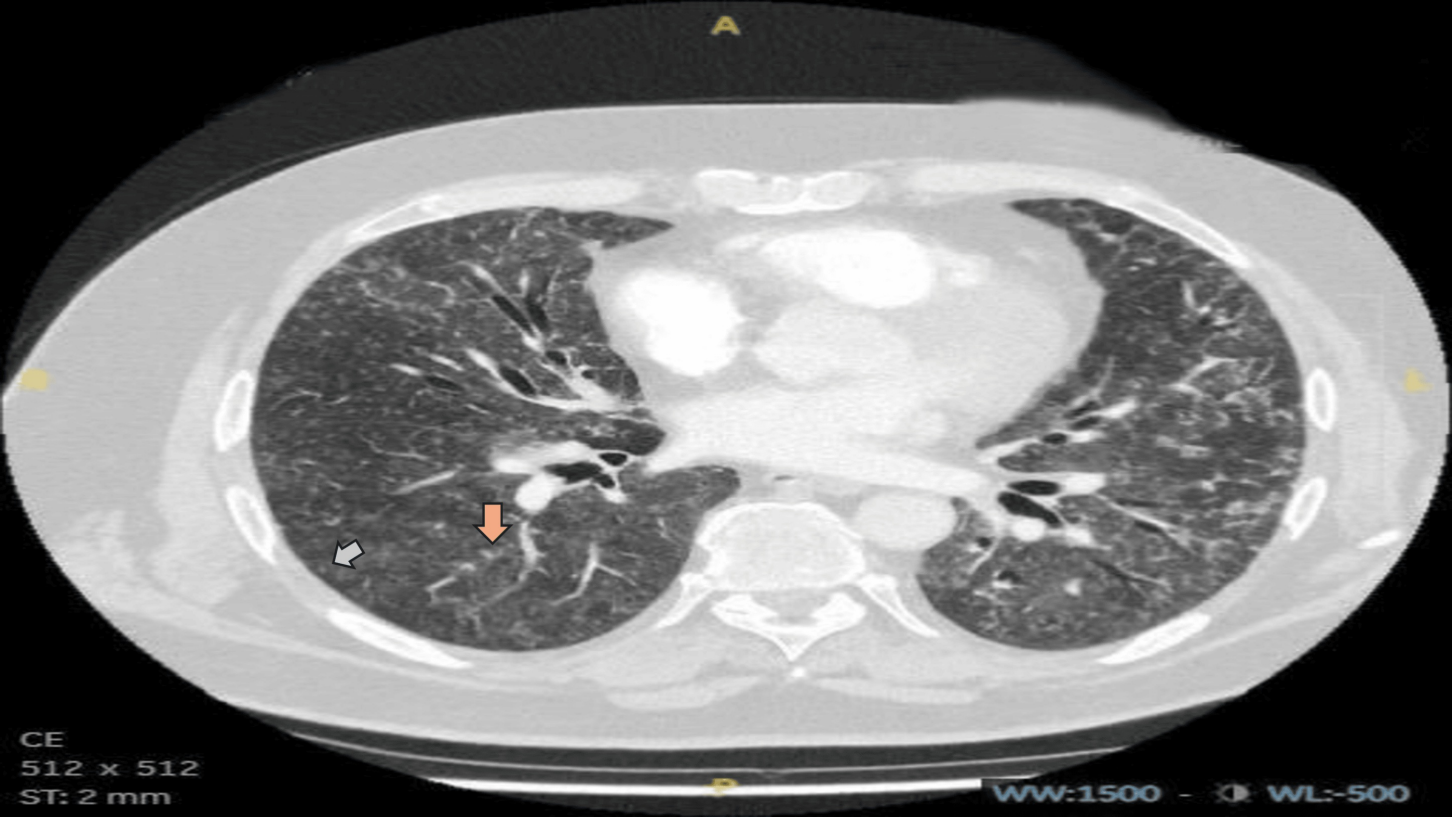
CTPA from current admission Initial CTPA revealed diffuse ground-glass opacities with a centrilobular nodular pattern in both lungs. Additional findings include mosaic attenuation, a tree-in-bud appearance, and areas of air trapping. The grey arrow indicates a centrilobular nodular pattern. The orange arrow indicates a tree in bud appearance. CTPA, computed tomography pulmonary angiography
